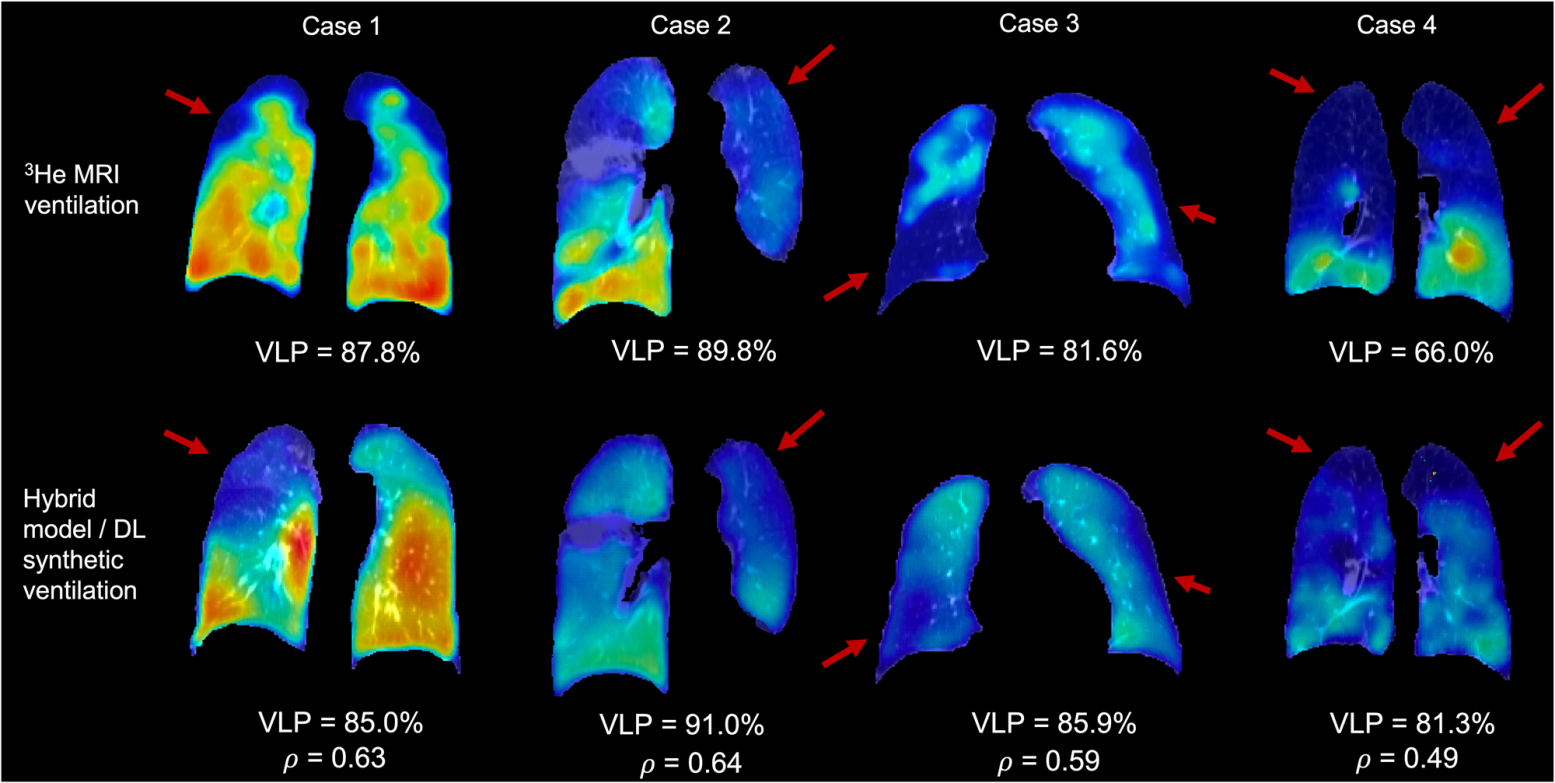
Fused ventilation (jet colormap showing minimum to maximum ventilation) and structural scans (grayscale) from four patients derived from either (top) ^3^He MRI and warped expiratory CT or (bottom) the proposed hybrid configuration and warped expiratory CT. All cases are from lung cancer patients with significant ventilation defects; red arrows indicate defects replicated in synthetic ventilation scans. VLP and Spearman's 𝜌 values are given.
